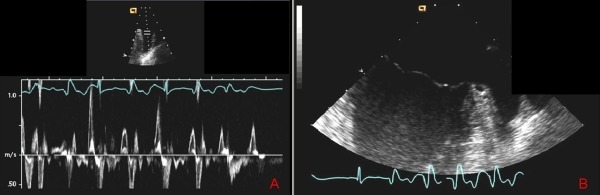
Transesophageal echocardiogram with LAA flow velocities (A) and multi-lobulated LAA (B) showing an almost 50% decrease in flow velocity during the PVCs and normal velocity during sinus rhythm (third and fifth beats) without evidence of intra-cardiac thrombus or spontaneous echo-contrast.
